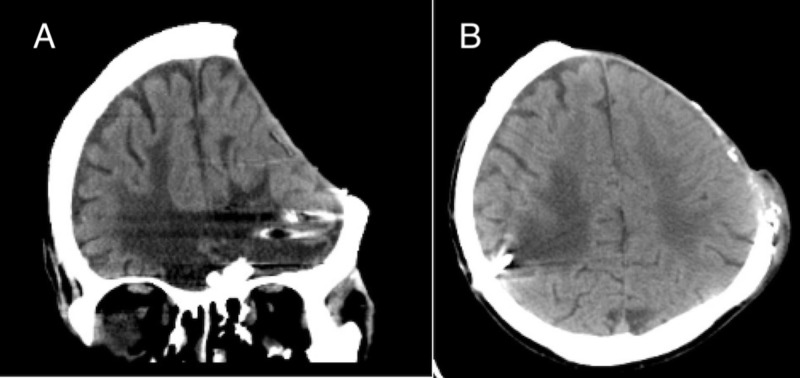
CT images of the patient. In each pane, the patient has radiologic evidence of syndrome of the trephined. However, the presence of this phenomena was difficult to determine clinically as many of his symptoms could also have been secondary to his prior, serious traumatic brain injury. (A) Coronal plane. (B) Axial plane.
